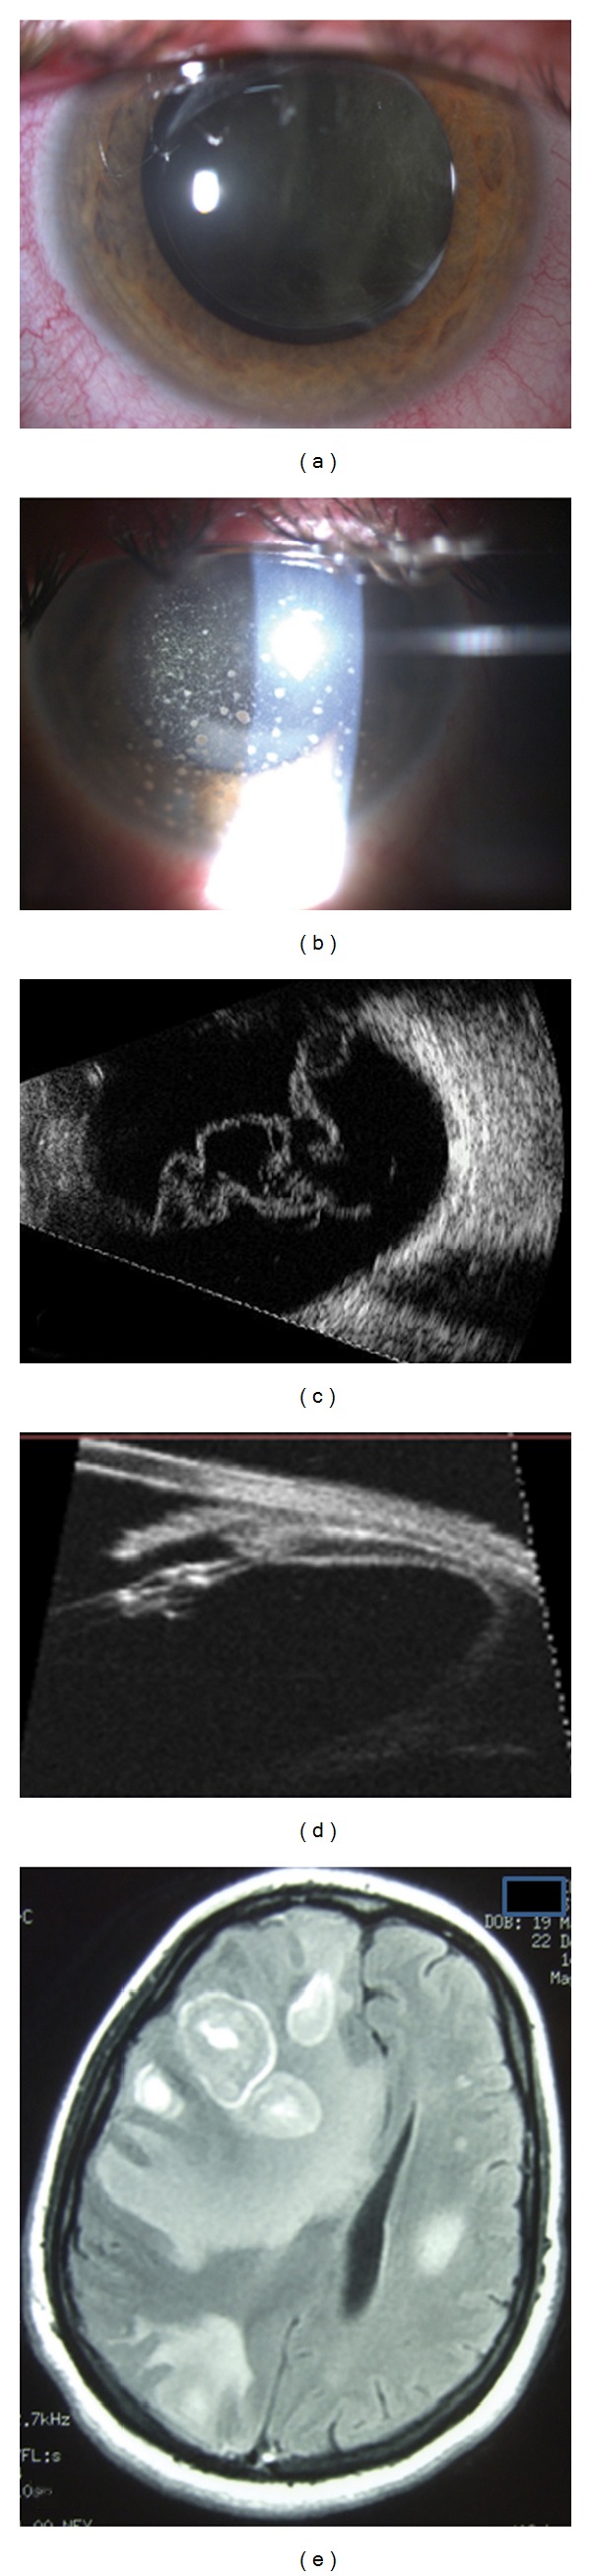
(a) Right eye showing a hazy vitreous humor. (b) Left eye showing an intense inflammation of the anterior chamber, with corneal precipitates. (c) Echographic examination of the vitreous in the right eye. (d) UBM examination of the ciliary body showing vitreous membranes adherent to the pars plana. (e) MR image showing a hypointense multifocal lesion occupying much of the right frontal and temporal lobe, the right occipital lobe, and the left parietal lobe.
